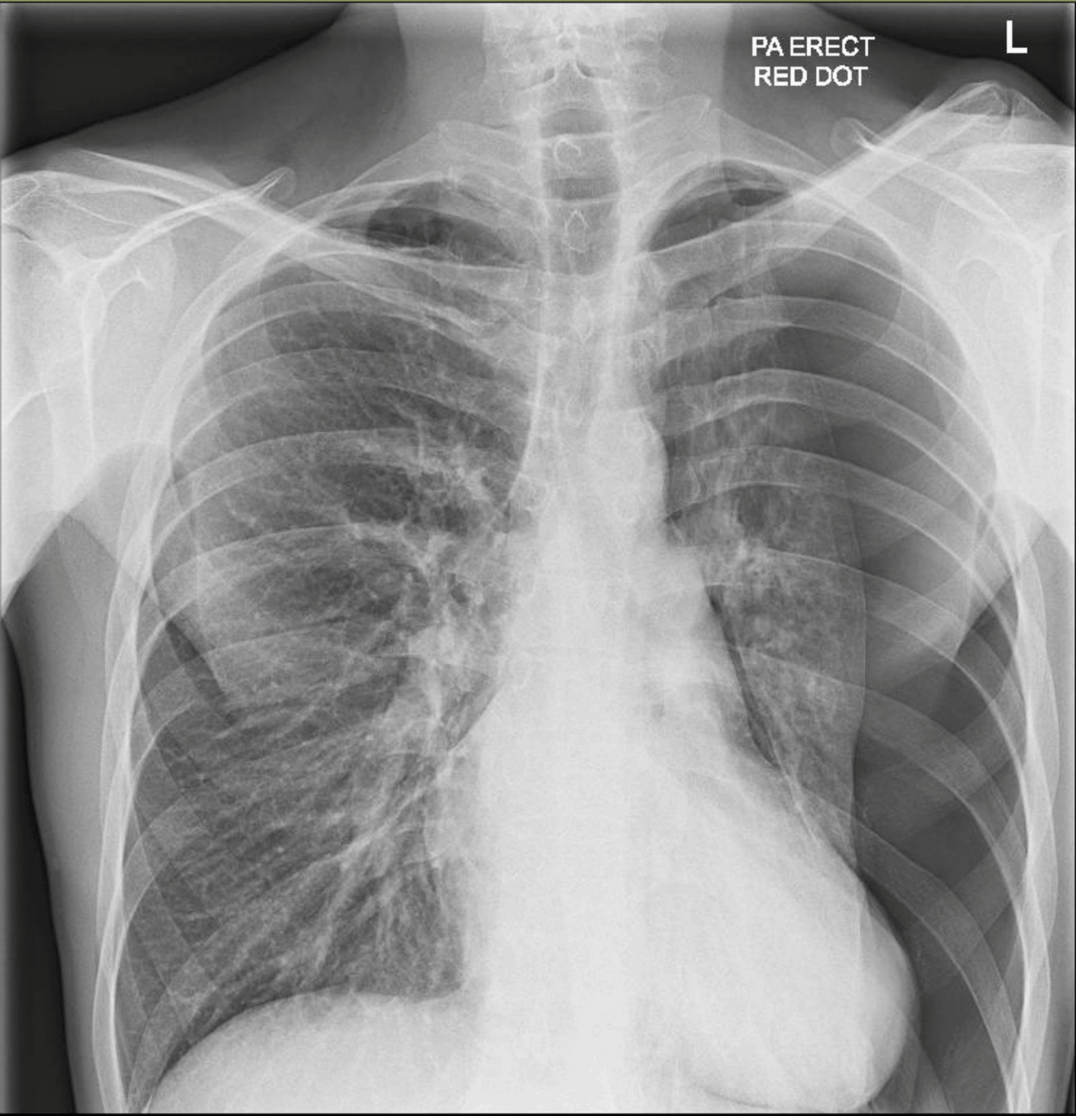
Chest X-ray on arrival showed a large left-sided pneumothorax
